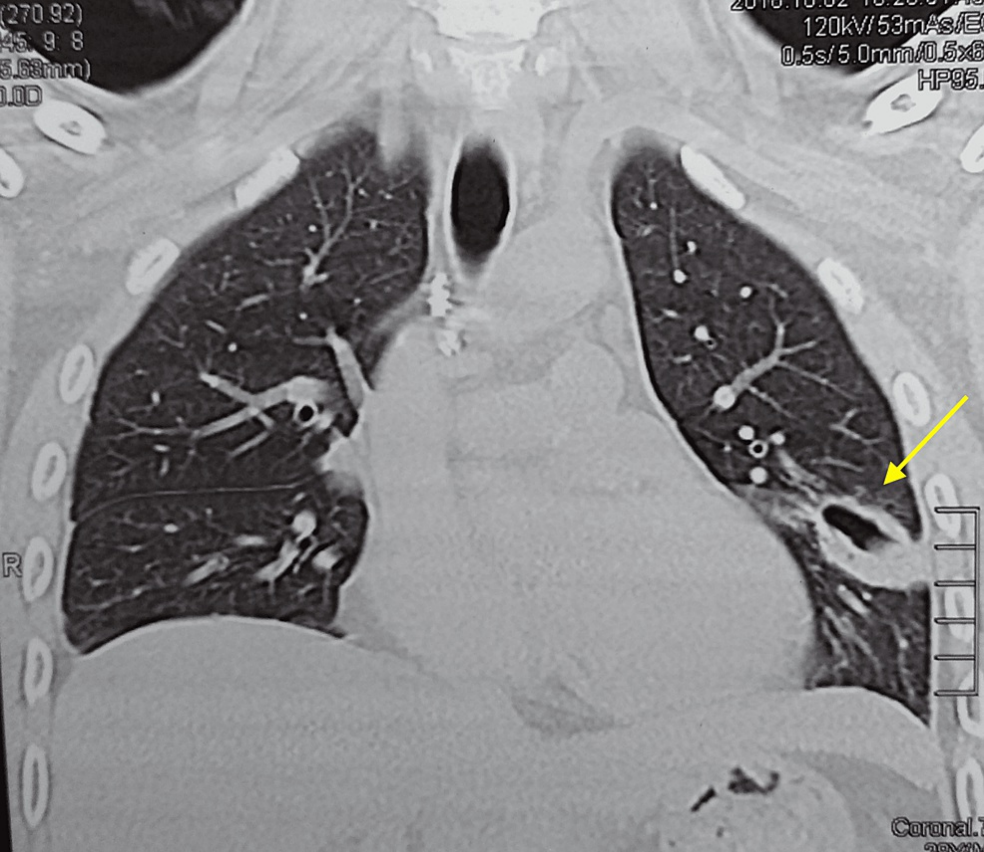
CT images revealed a 2.6 x 3-cm lingular cavitated lesion with thick walls, as well as fibrous tracts towards the visceral pleura CT: computed tomography
